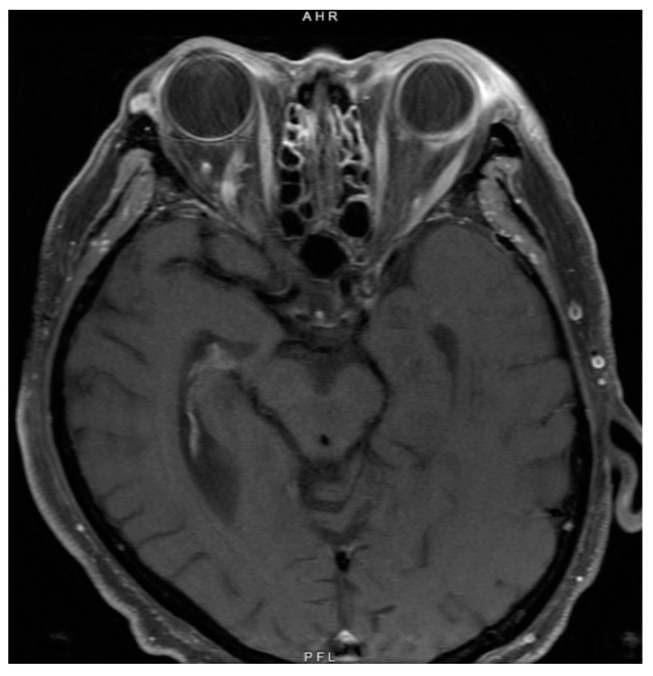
Magnetic resonance imaging of the brain and orbit at the time of initial diagnosis showed abnormal thickening of the left sclera and periorbital soft tissues but no lesions infiltrating the optic nerve to suggest malignancy.
